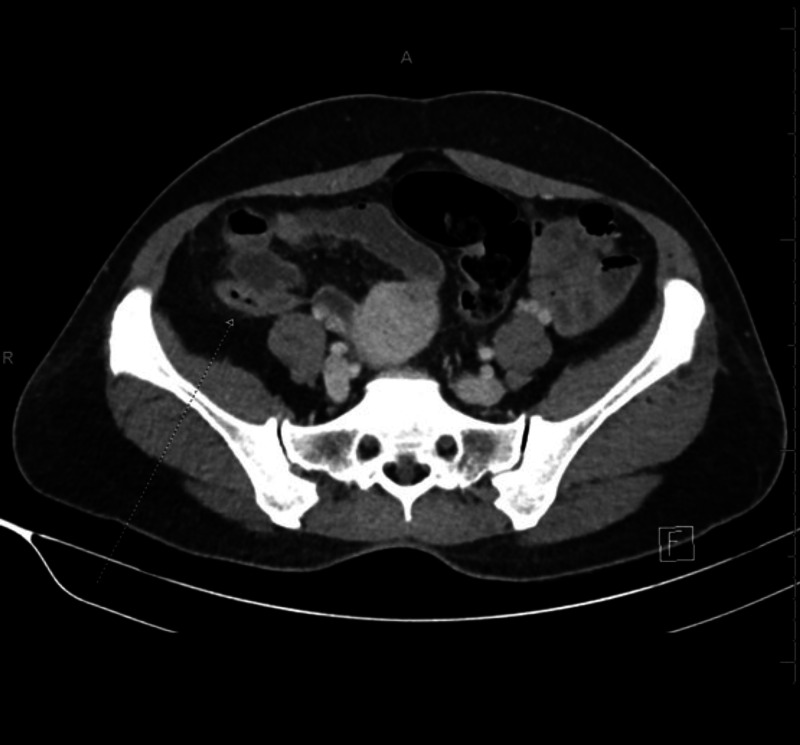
Axial enhanced computed tomography scan of the abdomen showing an inflamed appendix in the right iliac fossa (long arrow).
